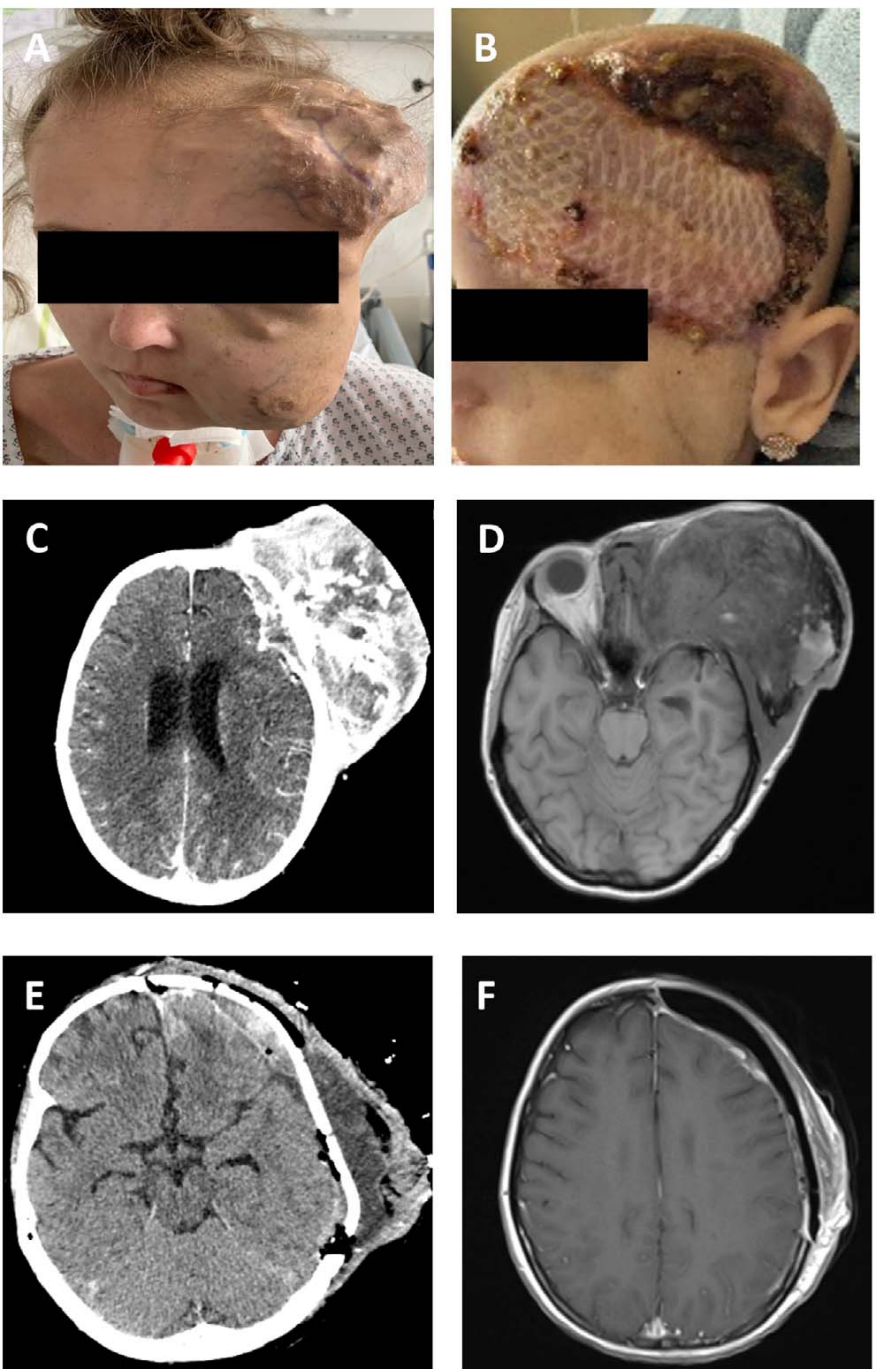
(A) Photograph shows tumor volume reduction and the appearance of necrotic tissue after 4 cycles of chemotherapy. (B) R0 resection was achieved with a successful reconstruction of the calotte. (C) Cranial computed tomography (CT) and (D) cranial magnetic resonance imaging (MRI) show significant shrinkage of the primary tumor (85 mm × 87 mm × 124 mm) after 4 cycles of chemotherapy. (E) Cranial CT shows postsurgical hematoma and fluid retention without evidence of tumor mass. (F) Two months postoperative cranial MRI shows regression of hematoma and fluid retention.
